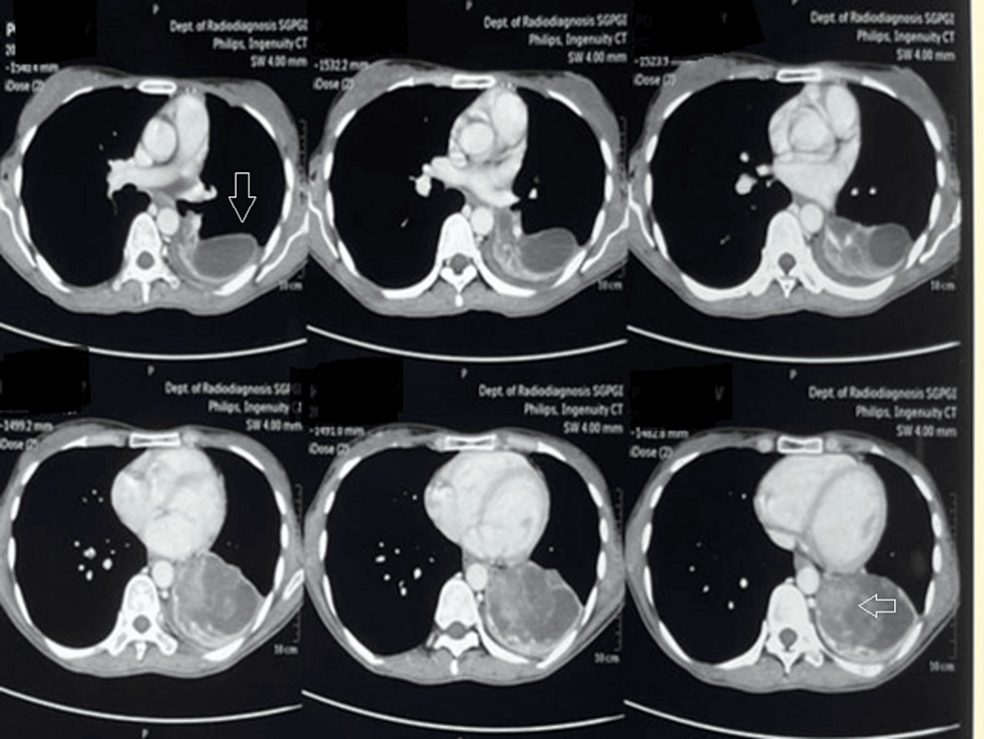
High-resolution computed tomography (HRCT) thorax showing an ill-defined heterogeneously enhancing lesion ~7.4 cm x 4.7 cm x 4.7 cm in the left lower lobe, infiltrating into the left lower lobe bronchus, with collapse of the left lower lobe (arrow)
